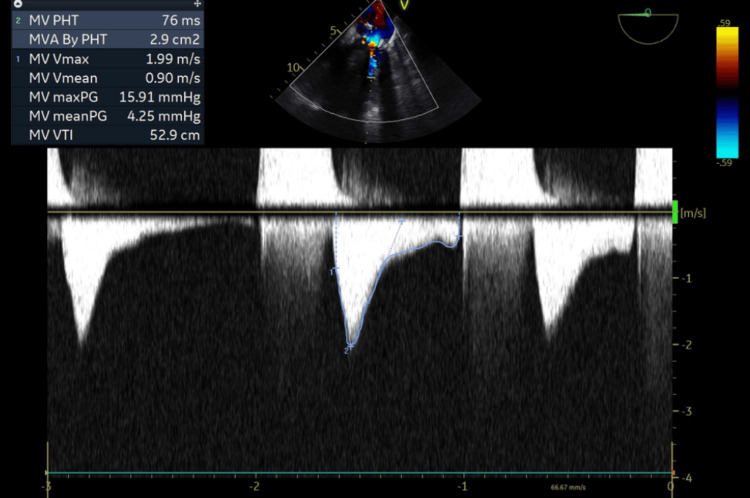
Transesophageal echocardiography showing mitral valve hemodynamics post Valve in Valve. Mean pressure gradient dropped to 4.25 mmHg.
